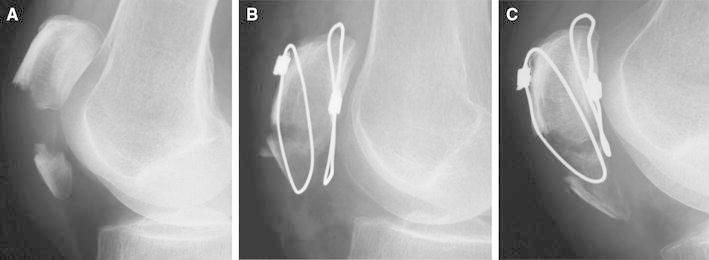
Displaced case (Case 5). a Preoperative plain X-ray radiography: AO type A, 2-part fracture. b The patellar tendon was not caught by the wire in the deep layer, and the fractured region was supported only by the wire in the shallow layer. c Plain X-ray radiography 4 months after surgery: the fractured region was displaced
